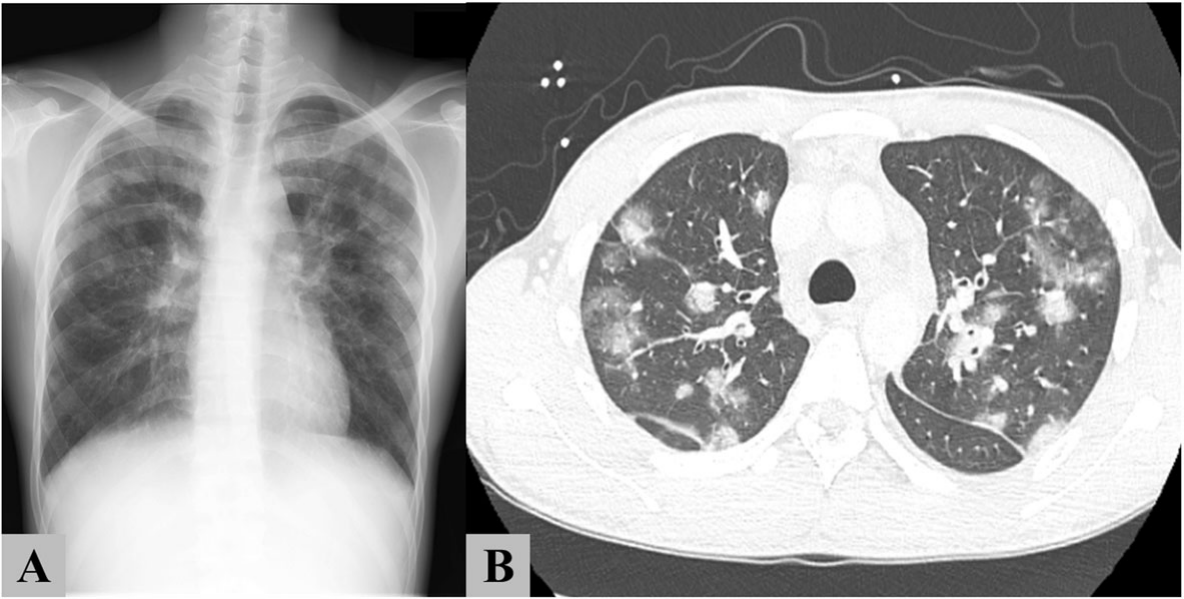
Radiological imaging on admission. Chest computed tomography revealed multiple nodules in both lungs that showed diffusely mixed with consolidation and ground-glass opacity pattern. (a; coronal section, b; horizontal section)
